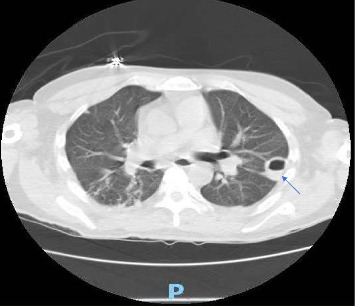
CT showing multiple cavitary lesions, opacities/nodules with peribronchial thickening. Suspicious for infection versus malignancy.
